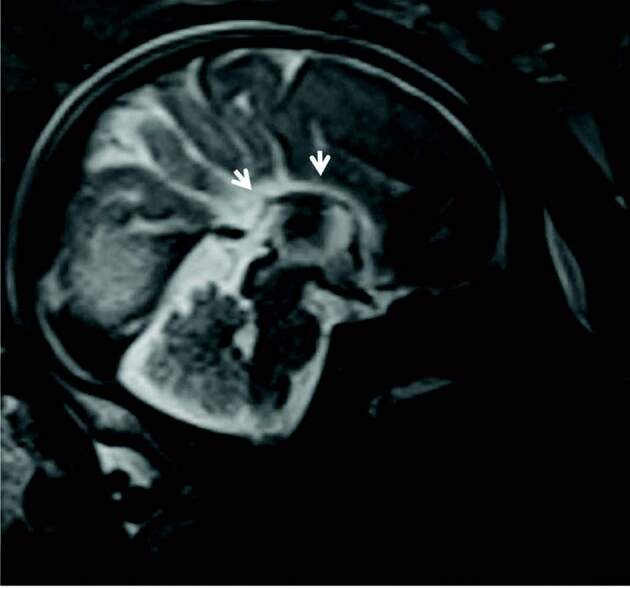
Sagittal T2 SSFSE fetal MR image demonstrates total agenesis of the corpus callosum.
